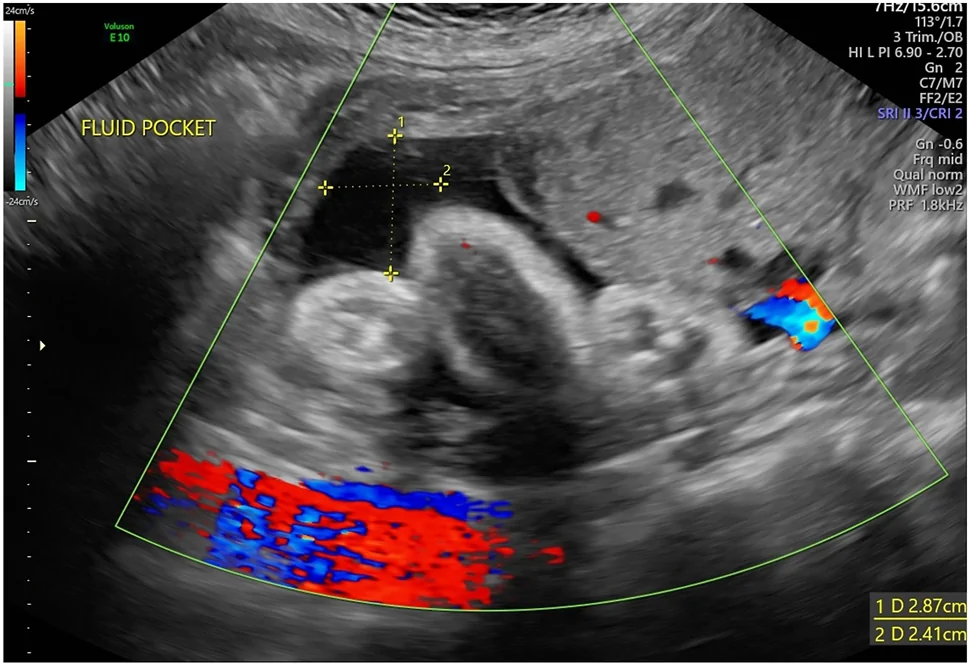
Ultrasound showing normal amniotic fluid.
